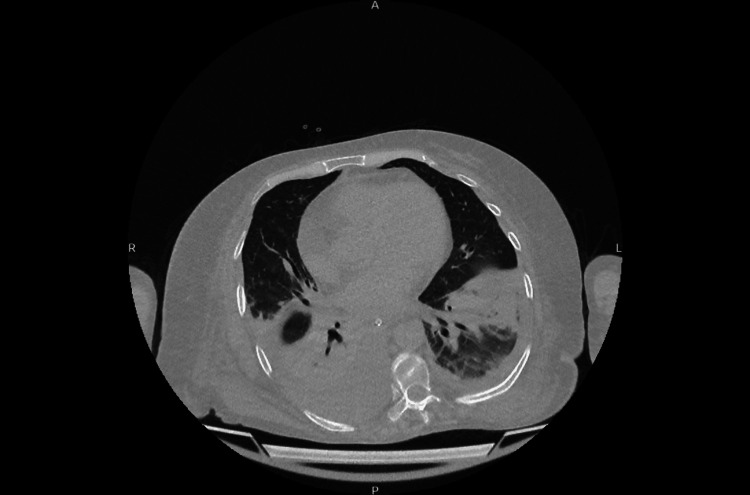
An axial computed tomography scan of the chest without contrast showing bilateral pleural effusions predominantly on the right and compressive atelectasis of the lower lobes, associated alveolointerstitial opacities, and inflammatory thickening of the chest wall extending from the pleural space
